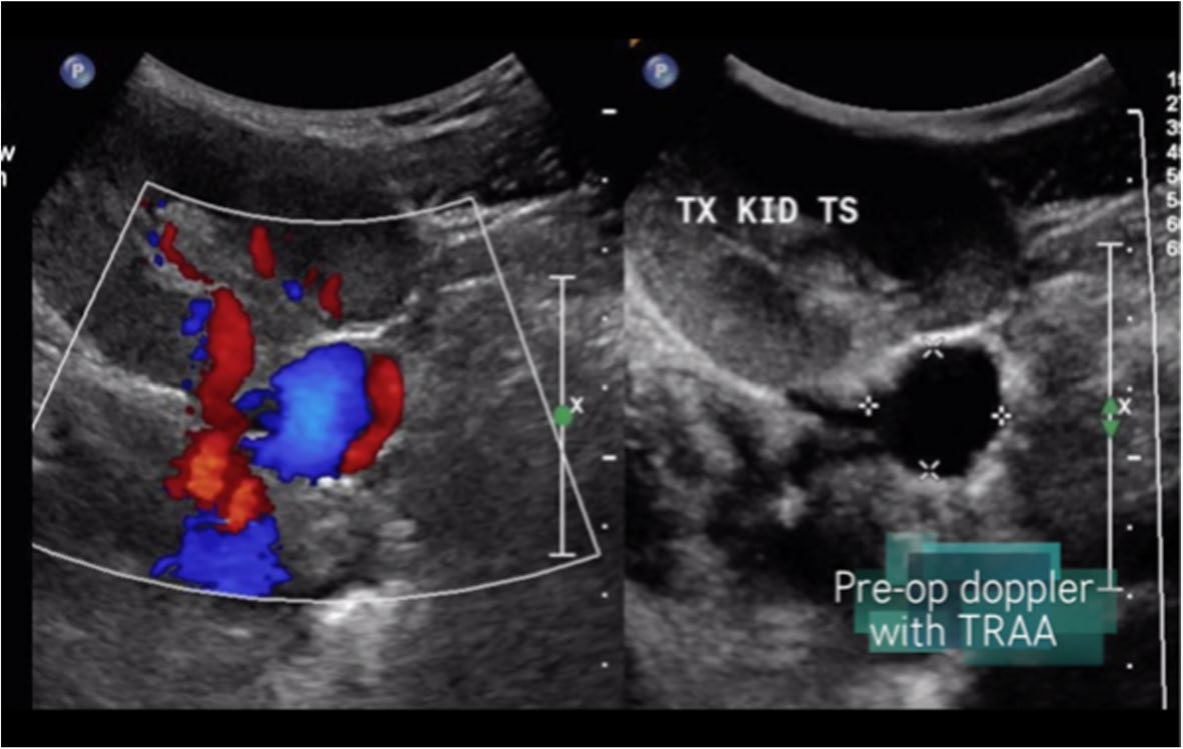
Ultrasound doppler showing TRAA
